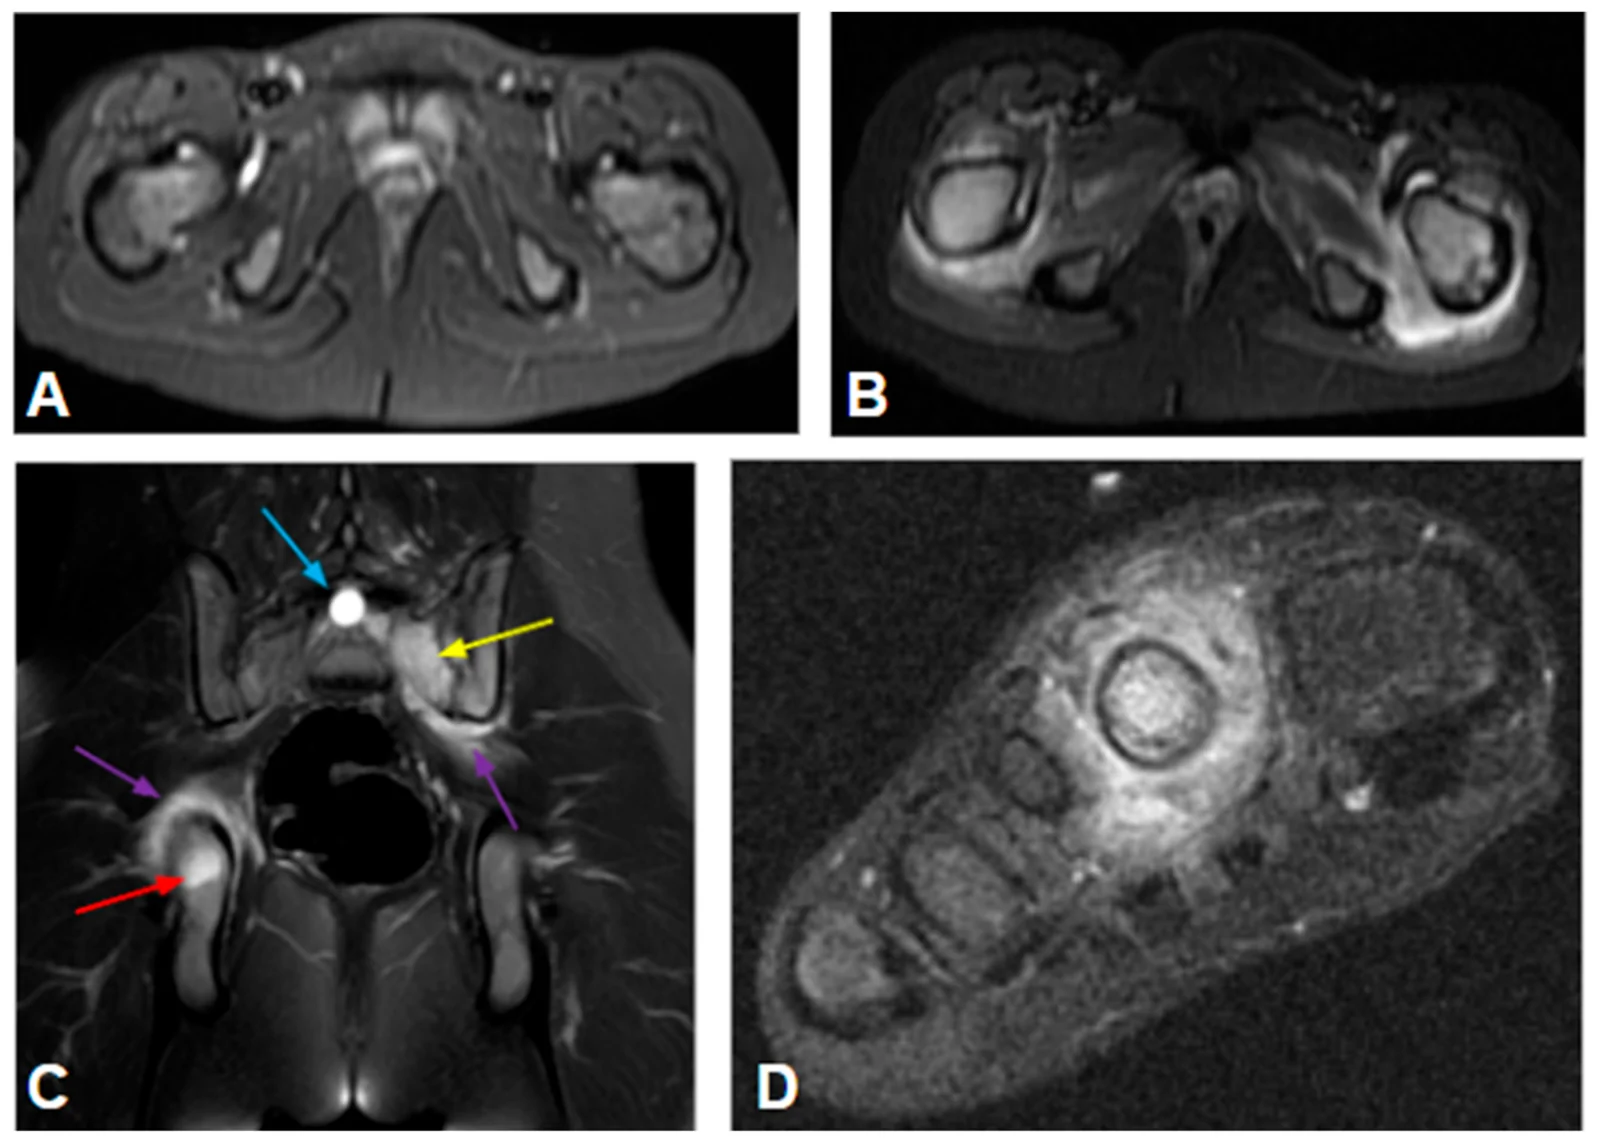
Pelvic and extremity magnetic resonance imaging (MRI) findings before and after treatment. (A) Patient 2; axial STIR image of the pelvis at 26 months of TNFi therapy: follow-up imaging demonstrates near-complete resolution of the prior BMH, with bilateral proximal femoral signals matching normal background bone marrow (ischial tuberosities and pubic symphysis), along with resolved soft- tissue hyperintensity. (B) Patient 2; axial short-tau inversion recovery (STIR) image of the pelvis prior to tumor necrosis factor inhibitor (TNFi) initiation: Marked bilateral proximal femoral bone marrow hyperintensity (BMH) tracking with fluid-equivalent signal intensity accompanied by extensive surrounding soft -tissue and muscular edema. (C) Patient 9; coronal T2 fat-saturated image of the pelvis: marked BMH within the right ischium (red arrow) demonstrating fluid-equivalent signal intensity (blue arrow), and moderate BMH within the left sacrum (yellow arrow) measuring below fluid signal. Surrounding soft-tissue edema is present (purple arrows). (D) Patient 1; coronal STIR image of the right foot prior to TNFi initiation: intense BMH within the second metatarsal demonstrating fluid-equivalent signal, with marked surrounding soft-tissue edema.
